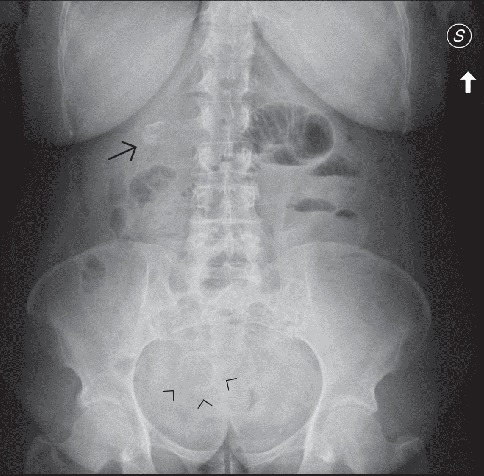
Upright abdominal radiograph shows the incomplete manifestation of the classical Rigler's triad in a patient with gallstone ileus. Note the paucity of intestinal gas, with dilated small bowel loops in the left upper abdomen and air-fluid levels. A stone with a thin peripheral calcified rim is visible (arrowheads), but pneumobilia is not detectable. Note curvilinear areas of increased opacity in the right upper quadrant, projecting into the gallbladder fossa (arrow).
